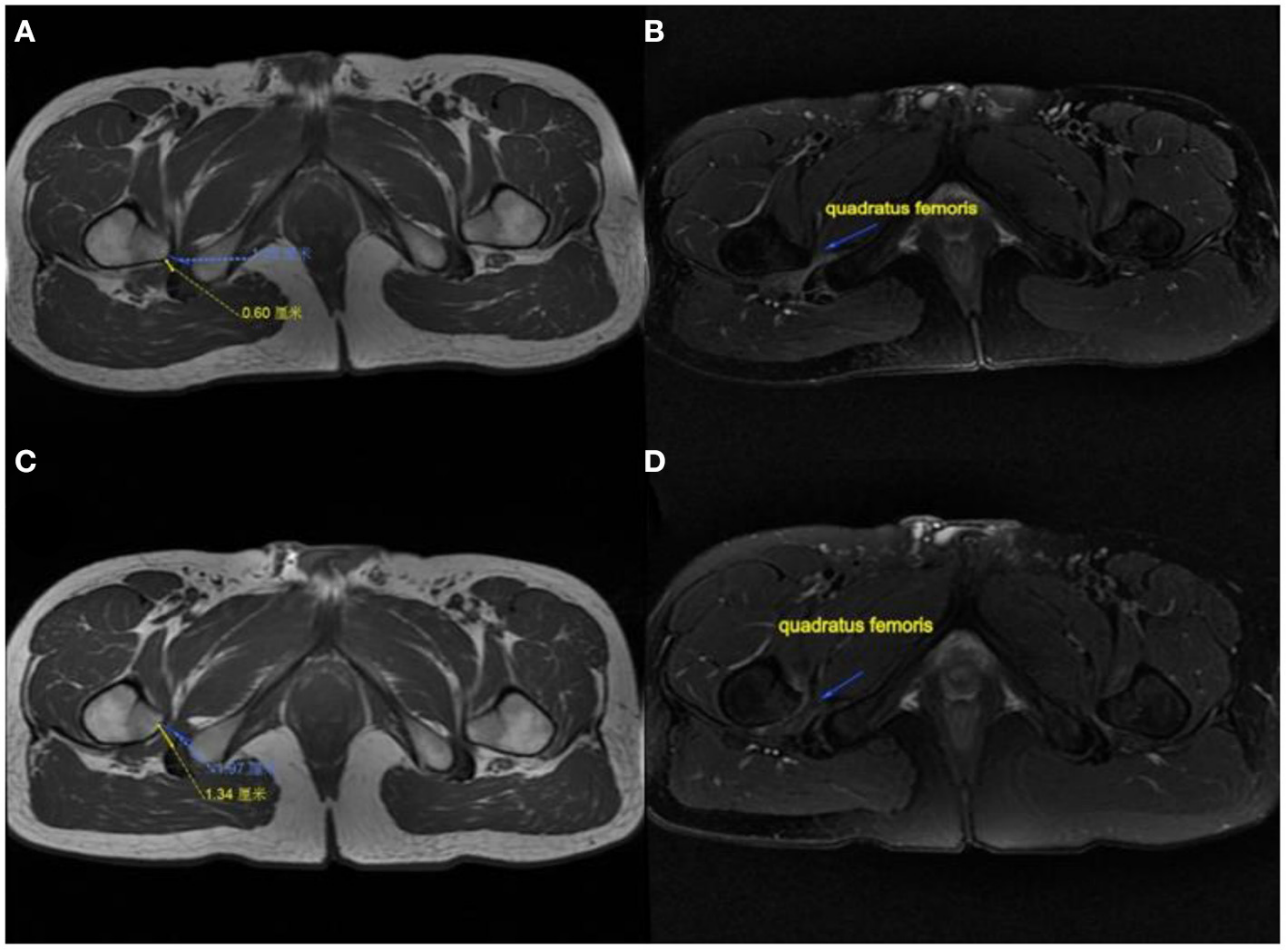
MRI performance of the affected hip in a patient. (A) IFS and QFS preoperation show narrowed. (B) Quadratus femoris pre-operation showing atrophy and degeneration. (C) IFS and QFS at 6 months postoperation show enlargement. (D) Quadratus femoris signal returned to normal and increased in volume at 6 months postoperatively. IFS, ischiofemoral space; QFS, quadratus femoris space.
